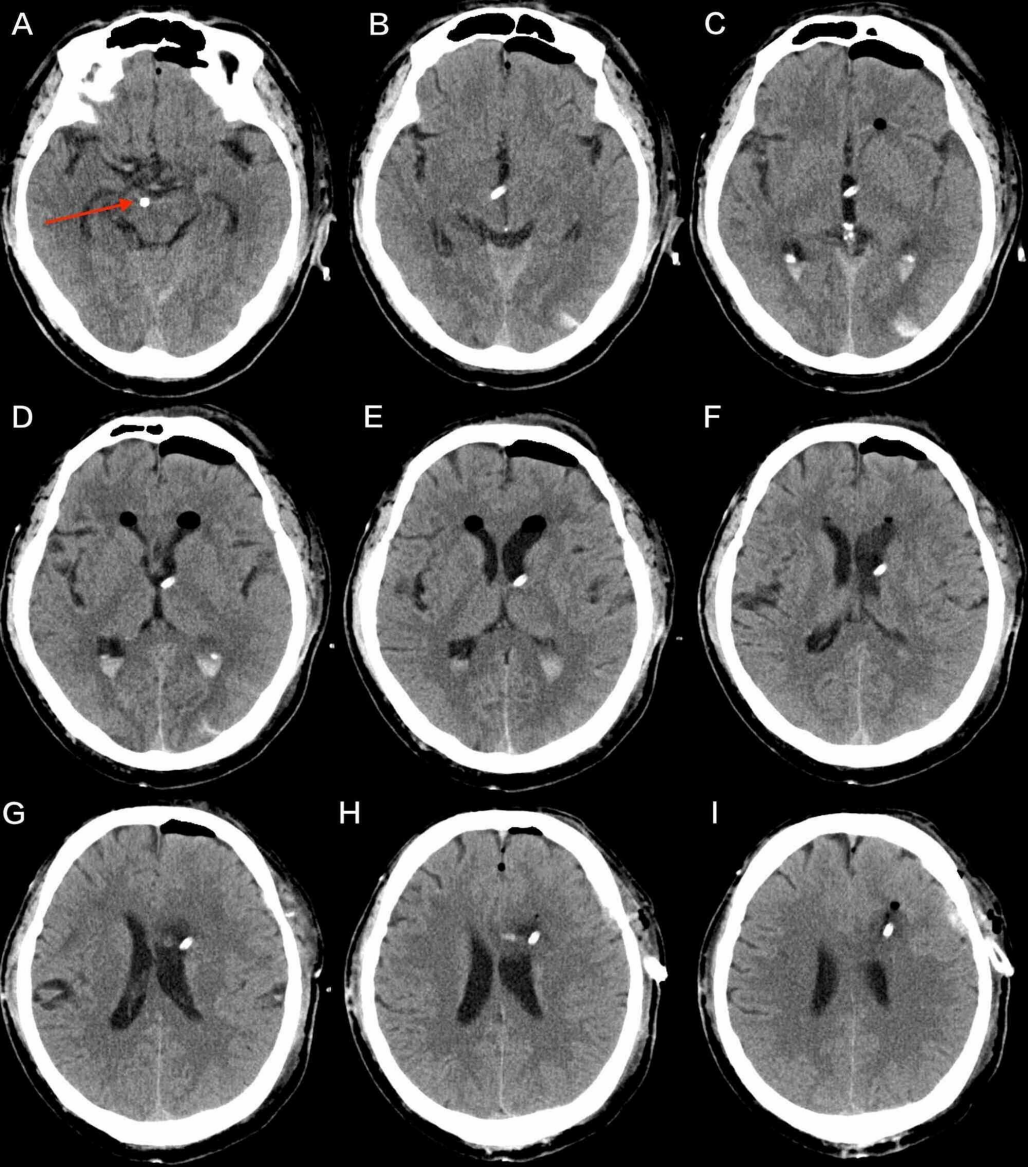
Axial CT scan (day 1 after the operation). Axial CT scan (day 1) revealing that the catheter traveled downward through the inferior parts of the third ventricle and reached the right cerebral peduncle (red arrowhead).
